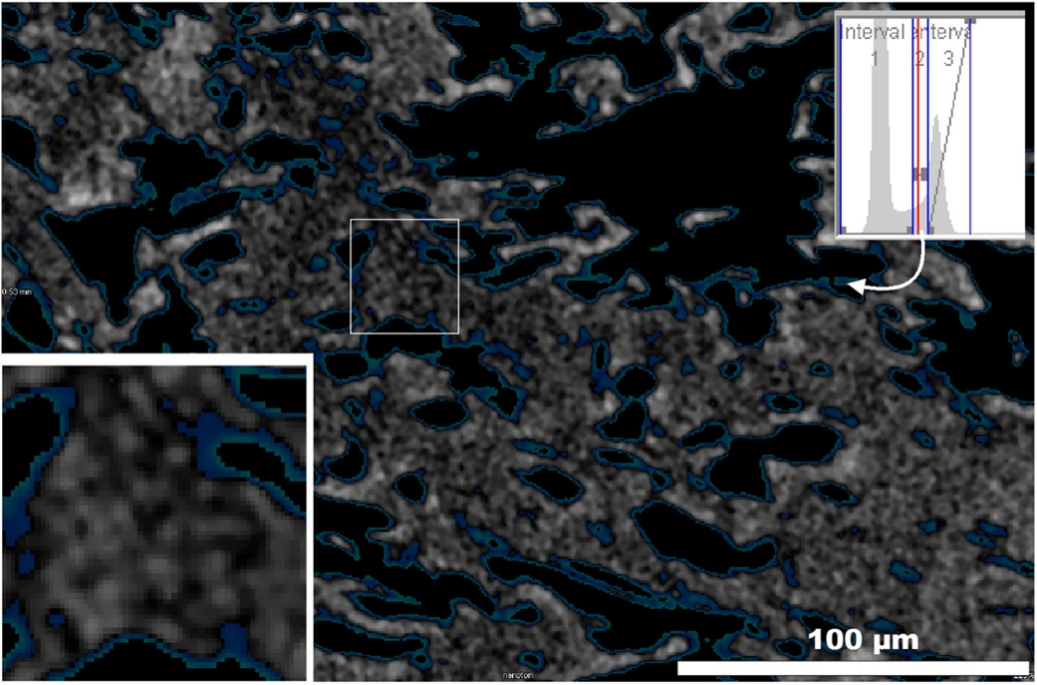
Grey value analysis of a CT section and magnified detail of the image. The top right insert shows the grey value distribution over the entire sample.
